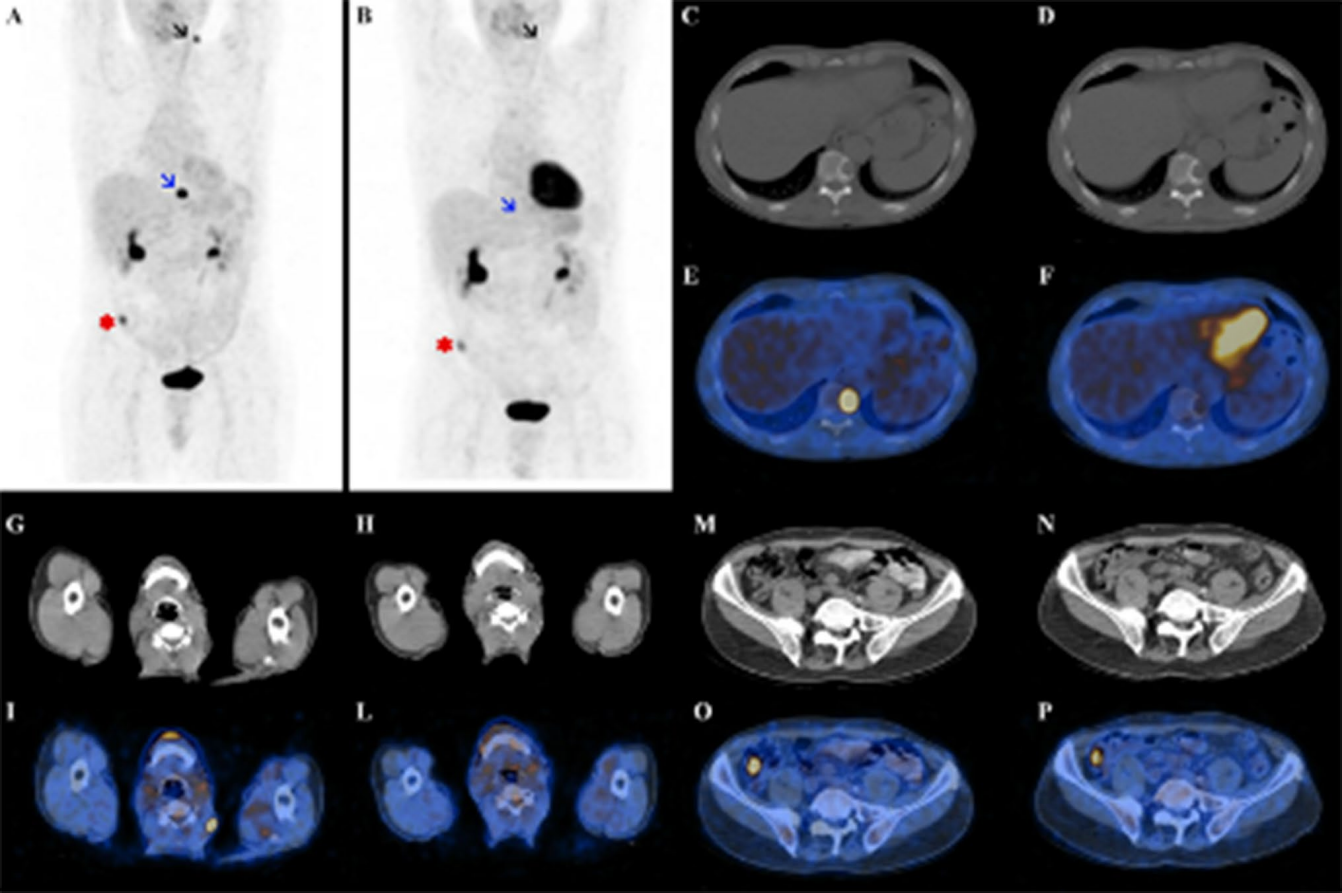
Case presentation of a 68-year-old male patient with metastatic PTC. He underwent total thyroidectomy (pT3mN1bMx) and two consecutive RAI treatments (cumulative activity 11.1 GBq). After the second RAI treatment, 18F-FDG PET/CT demonstrated three FDG-avid lesions: a left cervical lymph node (black arrow on a image; see also g and i), a lytic bone lesion in D10 (blue arrow on a image; see also c and e) and a focal intestinal uptake (asterisk on a image; see also m and o). So, the patient underwent a cervical lymphadenectomy that confirmed the thyroid origin of the lesion, and a Cyberknife radiosurgery of the D10 bone lesion (24 Gy). The subsequent follow-up 18F-FDG PET/CT scan demonstrated a complete metabolic response of the D10 bone lesion (blue arrow on b image; see also f), even if its radiological aspect was unchanged on CT (d); moreover, no further pathological radiotracer uptake was detected in the left cervical region (black arrow on b image; see also h and l). The focal intestinal uptake was stable (asterisk on b image; see also n and p) and was diagnosed as an adenomatous polyp at the subsequent polypectomy. The 18F-FDG PET/CT findings were further validated by the concomitant reduction of serum Tg levels. *PTC* papillary thyroid cancer, *RAI* radioactive iodine, *18F-FDG PET/CT* 18F-fluorodeoxyglucose positron emission tomography/computed tomography, *Tg* thyroglobulin
